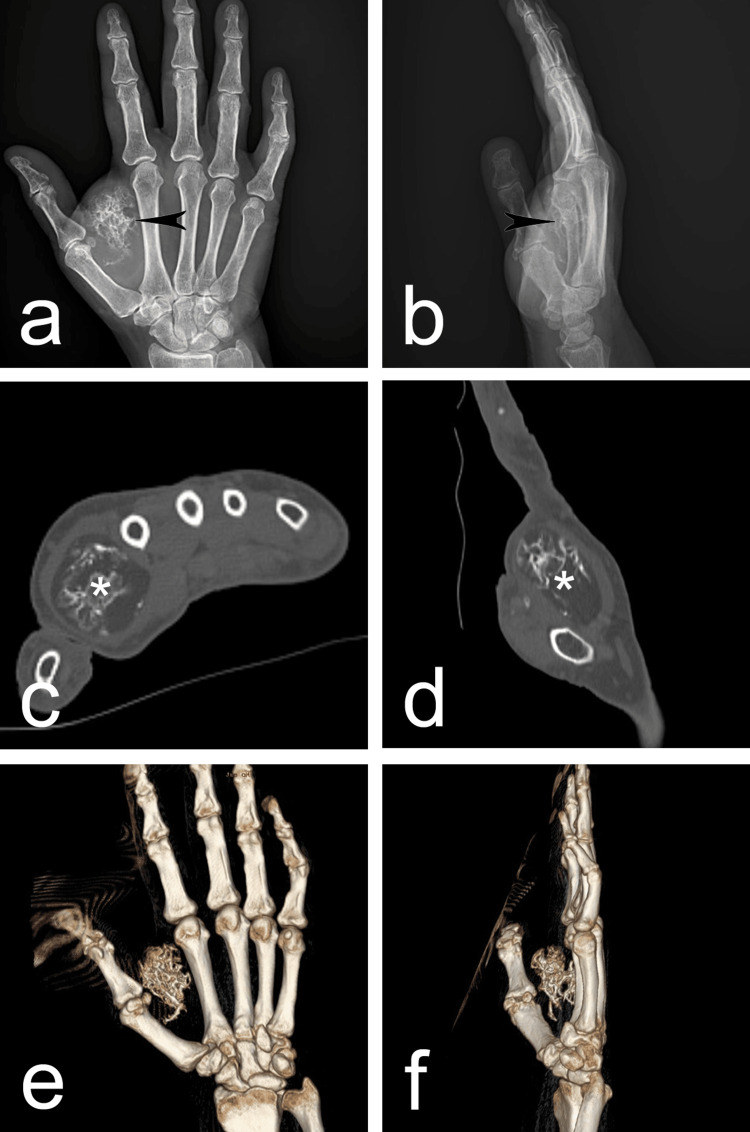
(a-b) Roentgenographies presented a clearly defined radiolucent ovoid formation soft-tissue mass with a trabecular calcified structure in it (arrowhead) in the space between the first and second fingers (a - anterior posterior view; b - lateral view). (c-d) CT imaging presented a soft-tissue mass with netlike calcification in its central part (asterisk) (c - transverse view; d - sagittal view). (e-f) 3D reconstruction CT: computed tomography; 3D: three-dimensional
